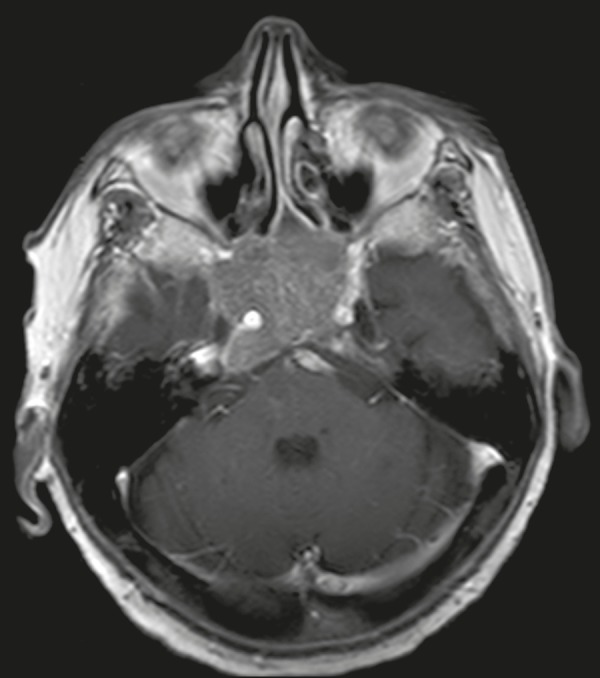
Contrast-enhanced axial T1-weighted MRI sequence showing a macroadenoma
